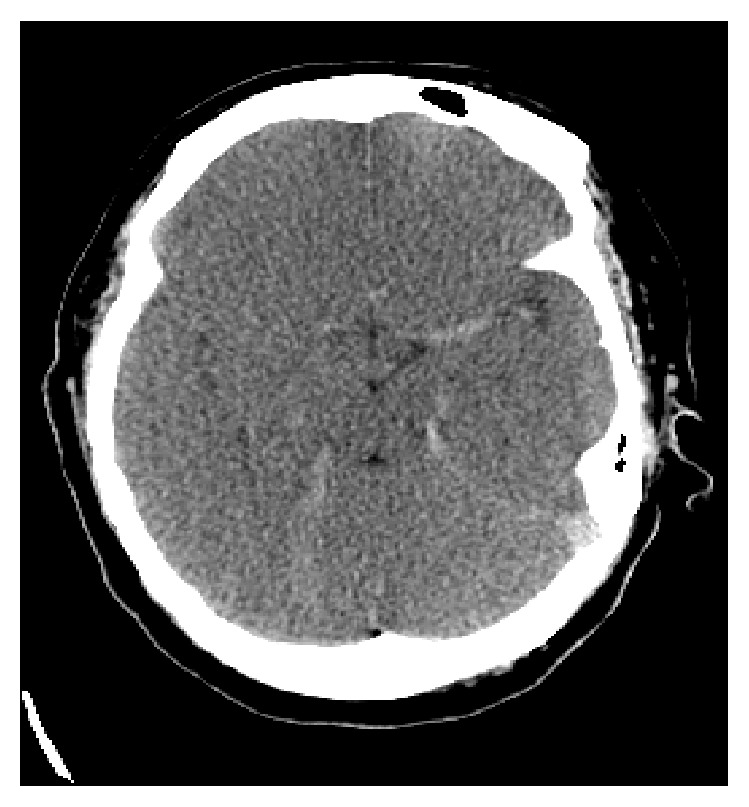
Noncontrast head computed tomography (CT) showing diffuse cerebral edema, with hyperattenuated cisterns and cerebral sulci.
